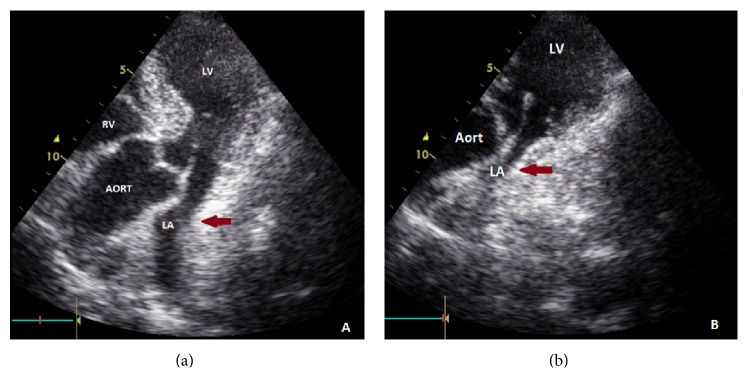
Transthoracic echocardiographic images of left atrial compression. Extrinsic compression to posterior wall of left atrium by hiatal hernia (red arrow). (a) Transthoracic echocardiographic view after fasting; (b) increased sol atrial compression on postprandial transthoracic echocardiography (red arrow); RV: right ventricle; LV: left ventricle; LA: left atrium.
